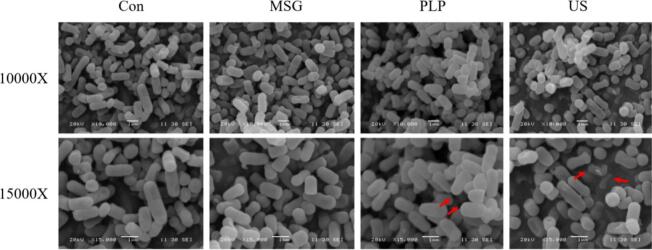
SEM images of *L. plantarum* JY7 cells: Control group (Con), L-MSG supplemented group (MSG), PLP supplemented group (PLP), and thermosonication group (US). Con: basal MRS; MSG: +0.5 % L-MSG; PLP: +0.5 % L-MSG + 45 μmol/L PLP; US: +0.5 % L-MSG + 45 μmol/L PLP, ultrasound (150 W, 25 min, 38℃).
